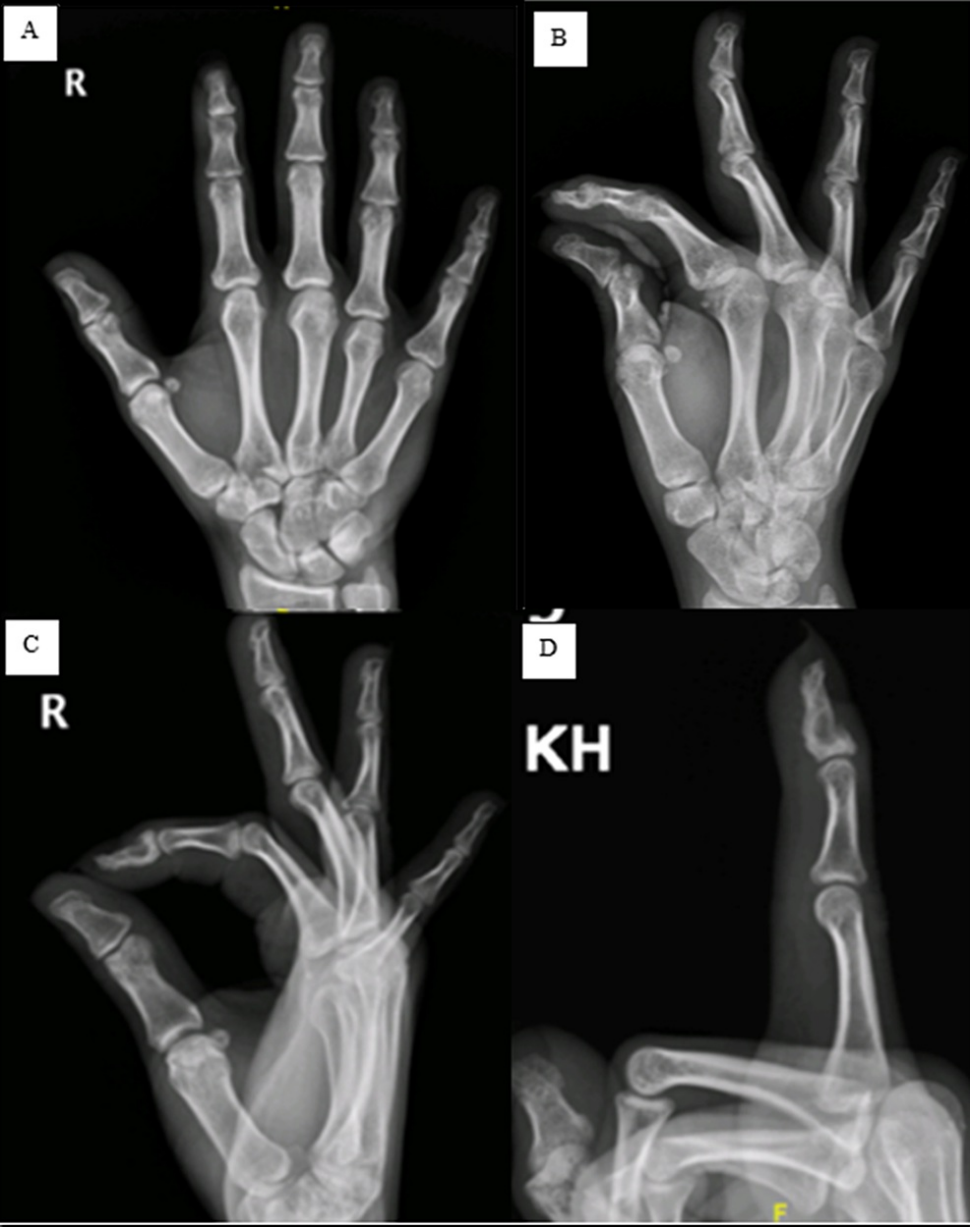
Follow-up radiographs at three months post-operative of the right-hand distal phalanx of the index finger showing no signs of fracture on (A) AP view, (B) oblique view, (C) lateral view, and (D) magnified lateral view focusing on the index finger. AP, anteroposterior.
